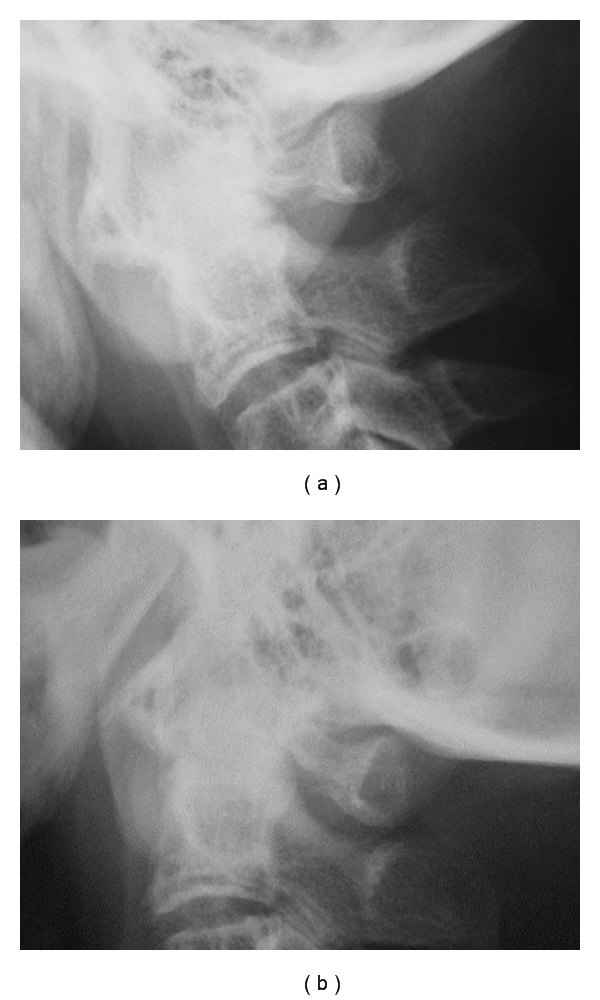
Preoperative dynamic lateral plain radiographs. Flexion view (a) and extension (b) view. Preoperative plain radiographs in flexed position (a) and in extended position (b) showed ossified mass behind dens and irreducible atlantoaxial subluxation. The atlantodental interval is 6 mm and the space available for the spinal cord is 7 mm in each positions.
